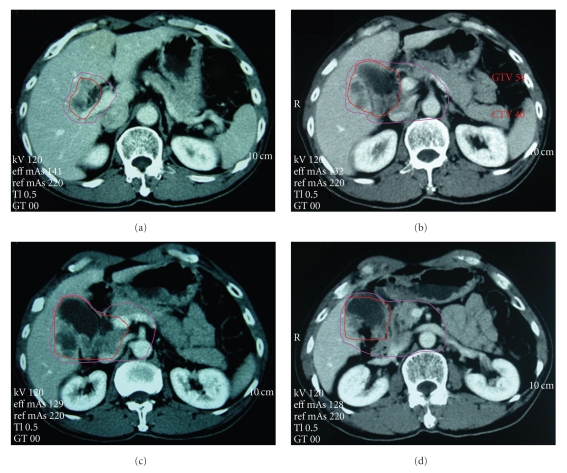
CT sections showing contours before radiotherapy. Radiation ports encompassed (a-b) regions of the liver involved by the gallbladder tumor and (c-d) carcinoma located at the neck of the gallbladder. GTV54 and CTV40 have been shown in the slice. Abbreviation: CTV: clinical target volume with pink lines; GTV: gross tumor volume with red lines.
